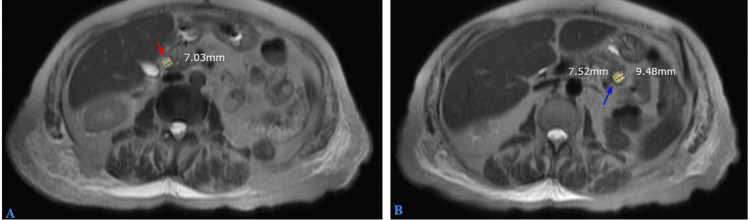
MRI with a pancreatic protocol showed the pancreas to be mildly atrophic with mild prominence of the pancreatic duct. A cyst was noted in the pancreatic head (A, red arrow) and an additional cyst in the pancreatic tail (B, blue arrow), likely representing a small IPMN. MRI, magnetic resonance imaging; IPMN, pancreatic Intraductal papillary mucinous neoplasm
